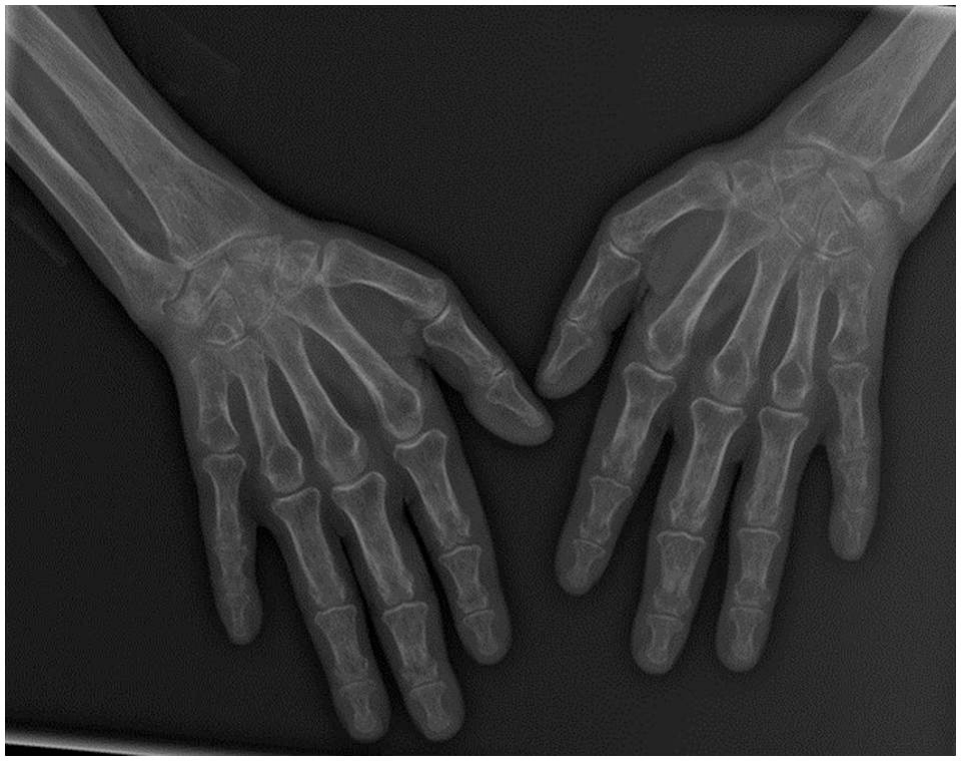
X-ray hand show an idiopathic isolated bilaterally and symmetrical brachymetacarpia of the fifth metacarpal bone.
